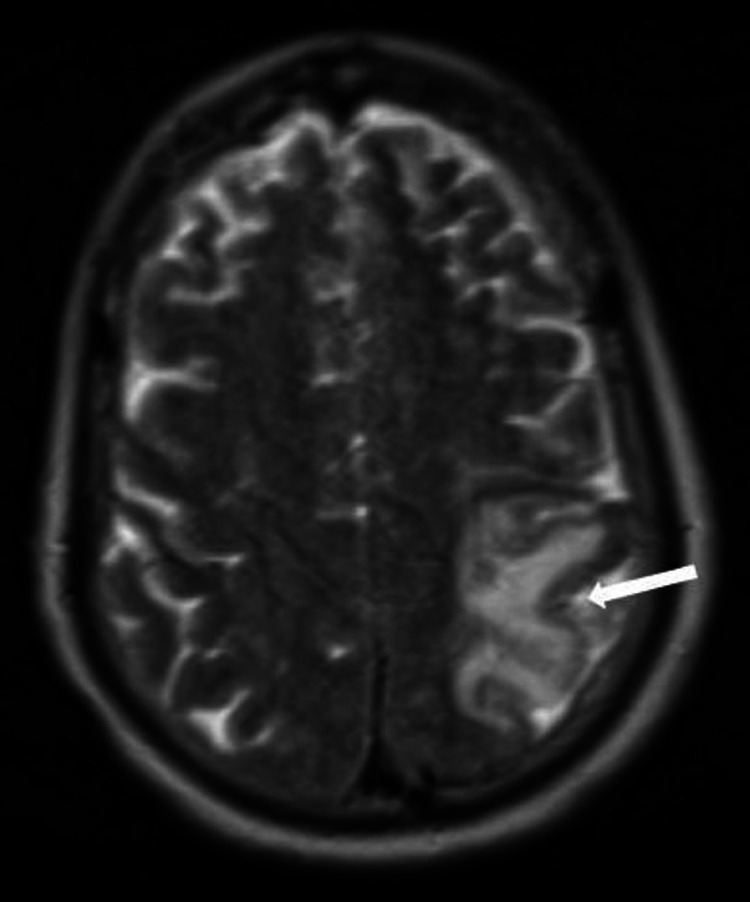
MRI brain with subacute infarct and associated edema (arrow) around left MCA. No evidence of hemorrhage or new acute infarct is demonstrated MRI: magnetic resonance imaging; MCA: middle cerebral artery
